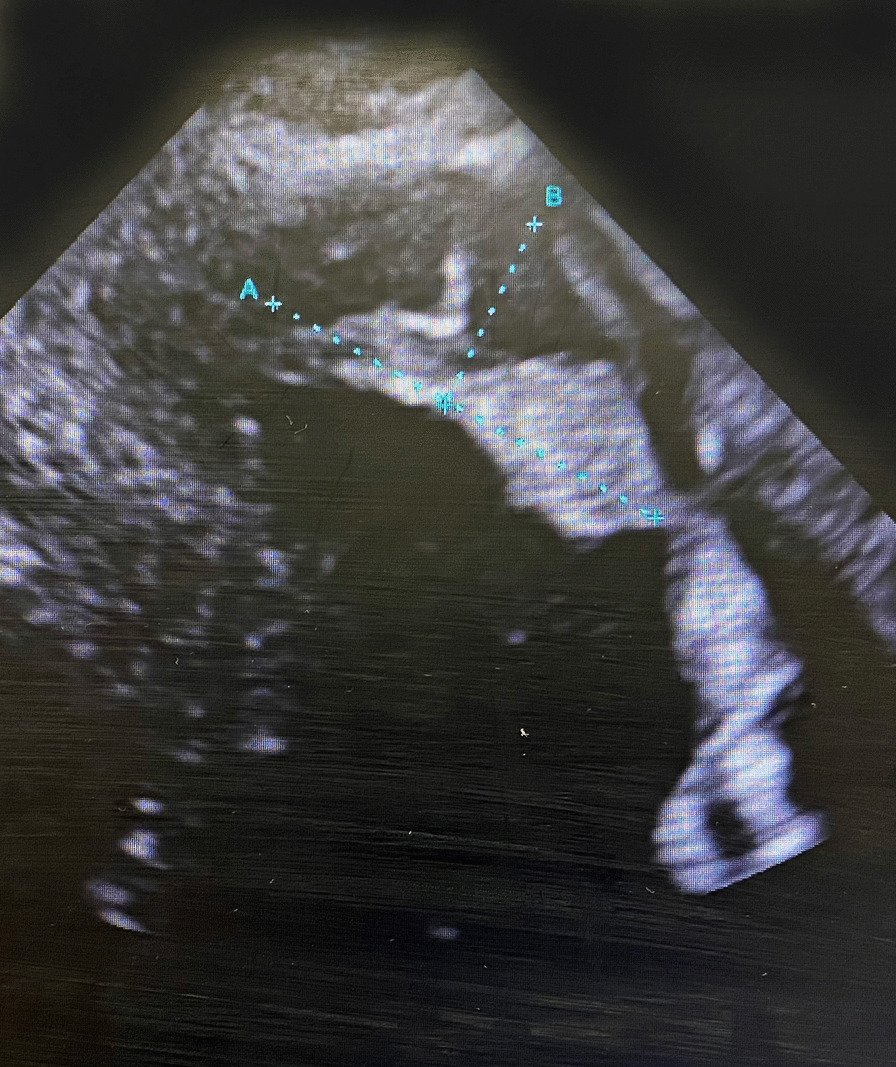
Preoperative echocardiography revealed a partially mobile thrombus at the LV apex
